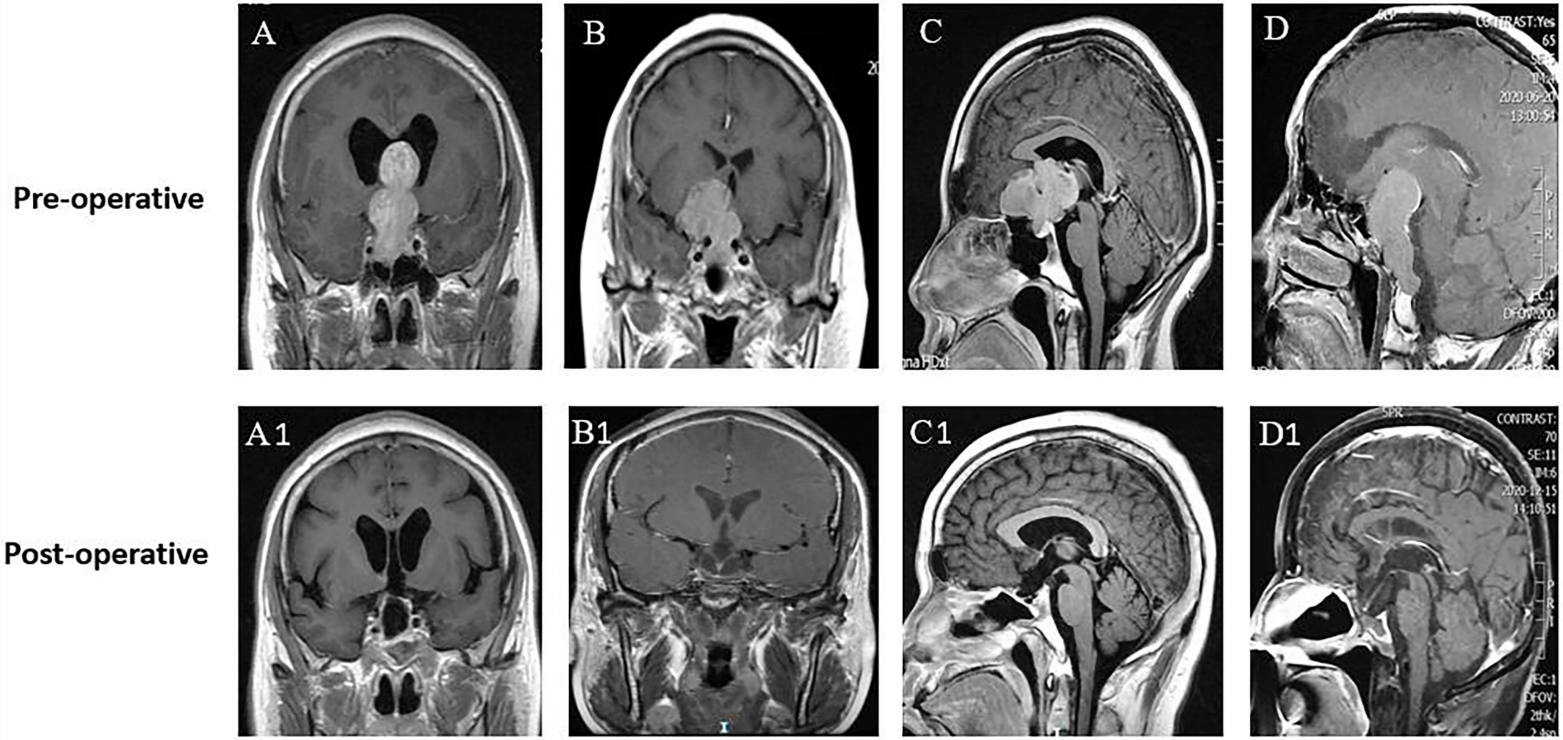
Different directions and extents of suprasellar extension did not influence the EOR. (A–D) Preoperative MR images of 4 patients with GPAs. All patients were characterized by no sign of cavernous sinus invasion (Knosp grade 0-I). (A) Gourd-like GPA with extreme suprasellar extension into the third ventricle and obstructive hydrocephalus. (B) Dumbbell-shaped GPA with lateral extension and displacement of the left anterior cerebral artery. (C) Lobulated GPA with forward extension into the planum sphenoidale. (D) Oval-shaped GPA with suprasellar and posterior fossa extension by dorsum sellae and clivus erosion. (A1–D1) Postoperative MR images of A–D, respectively, showing no visible residual tumor (GTR) in these GPAs of different suprasellar extensions.
